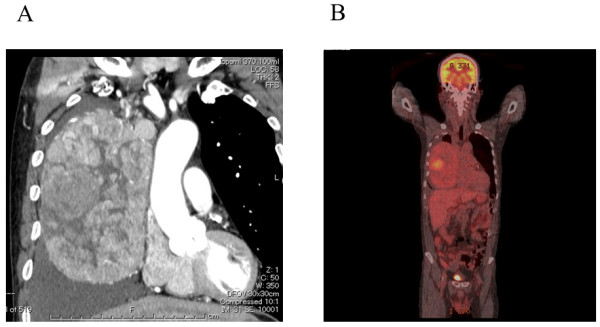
(A) Chest CT showing a huge mass, measuring 18 × 14 × 11 cm in diameter in the right thoracic cavity.(B) FDG-PET revealed that SUVmax of the tumor was 5.0 in the center and almost 2.5 in the surrounding areas.
